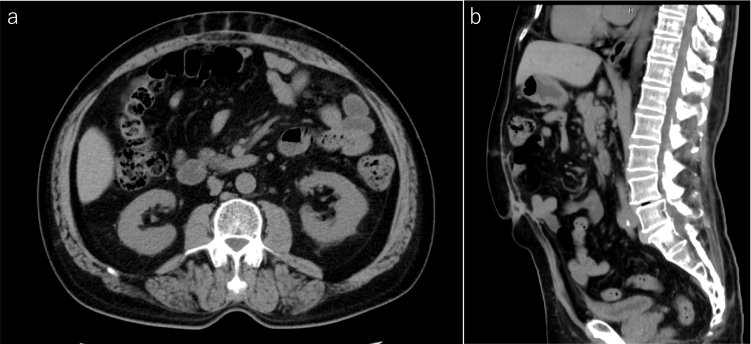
Abdominal computed tomography 6 months after interparietal hernia repair. There was no evidence of hernia recurrence, including interparietal hernias. a) and b) show the axial and sagittal images, respectively.
